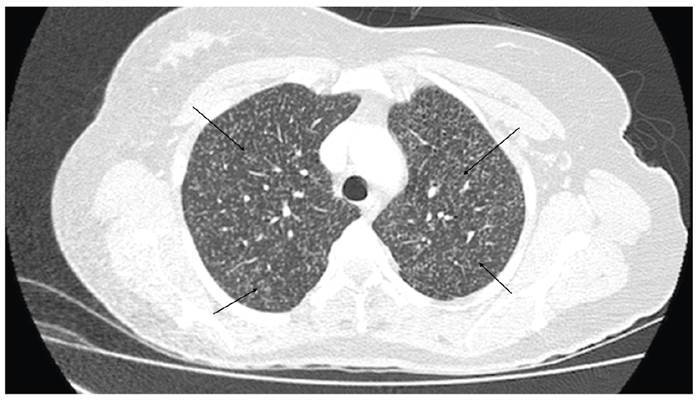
Chest computed tomography, with the axial pulmonary window showing multiple randomly distributed pulmonary micronodules (arrows) in both lungs.
